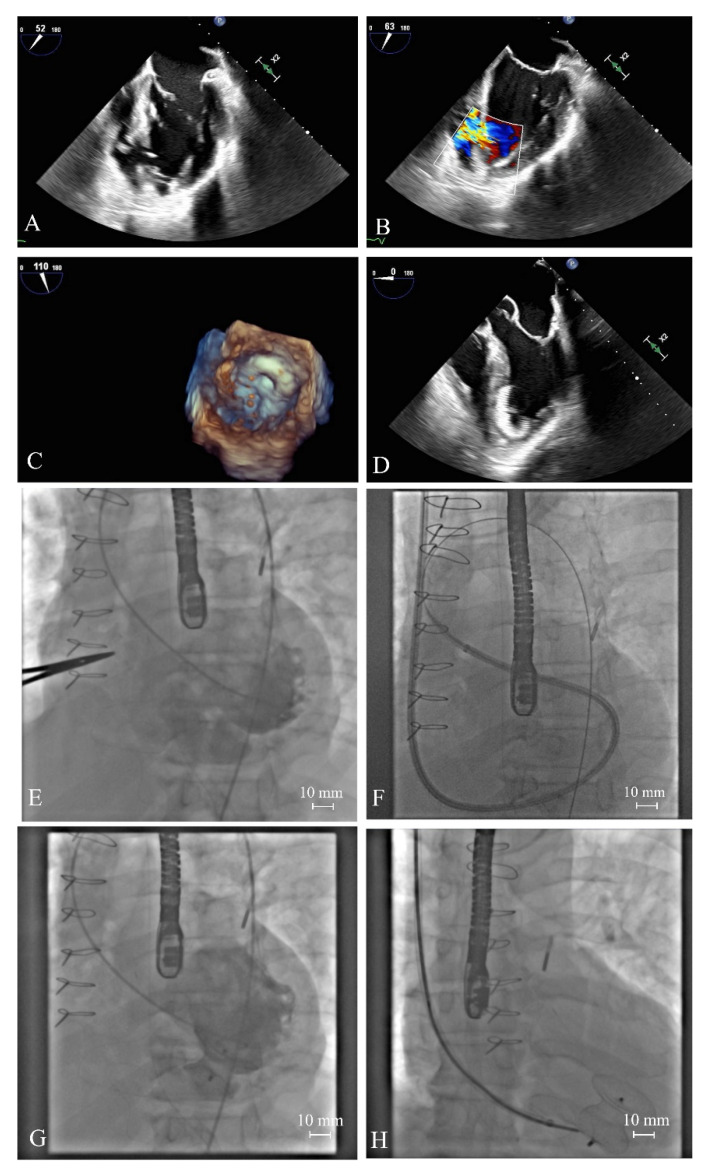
A patient with recanalized ventricular septal rupture initially treated with cardiac surgery. Ventricular septal rupture in transesophageal echocardiography (A,B) and LV angiography (E) treated with the Amplatzer occluder, inserted via the arteriovenous loop (F,G) with a nice effect (D,H). The LV surface of the Amplatzer occluder (C). LV: left ventricular.
